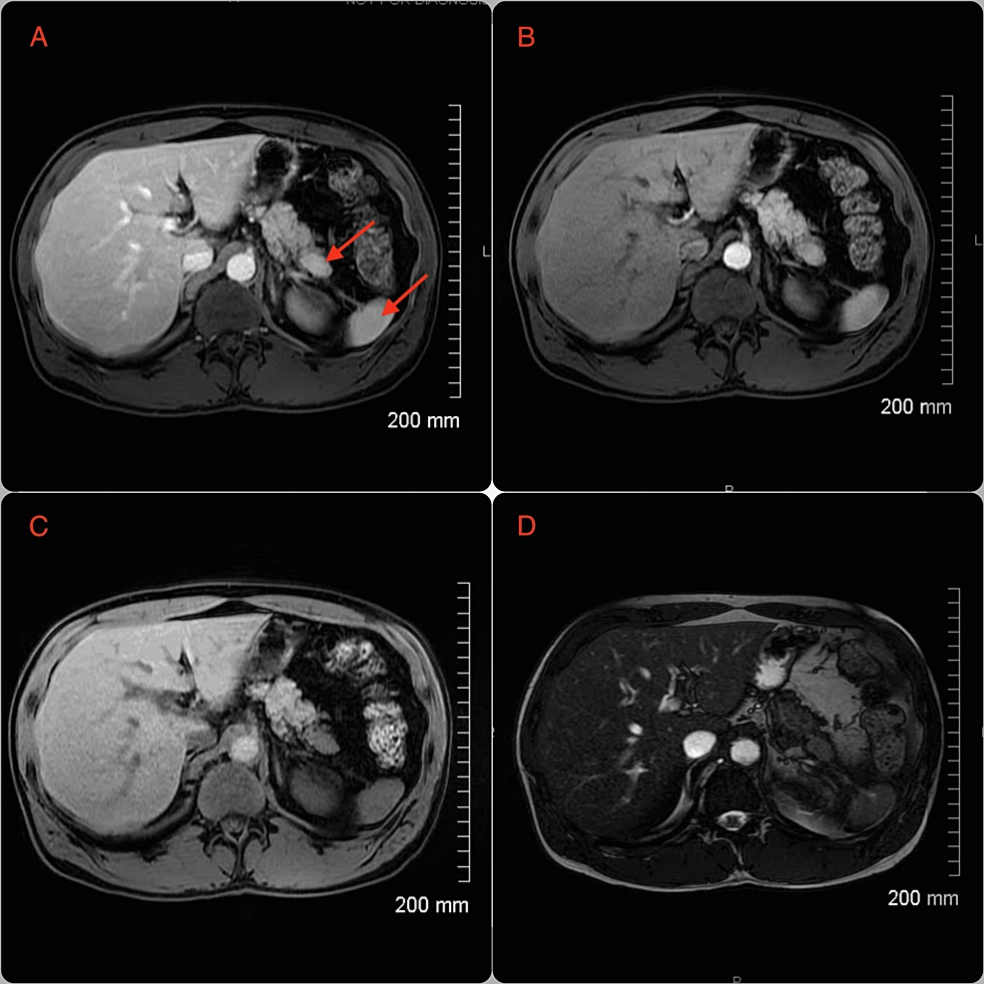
Axial MRI shows a 1.4 cm × 2.3 cm mass in the superior pancreatic tail isointense to the spleen with similar enhancement characteristics. (A) Pre-T1 fat saturated MRI, (B) arterial phase T1 MRI, (C) delayed phase T1 MRI, and (D) T2 MRI.
